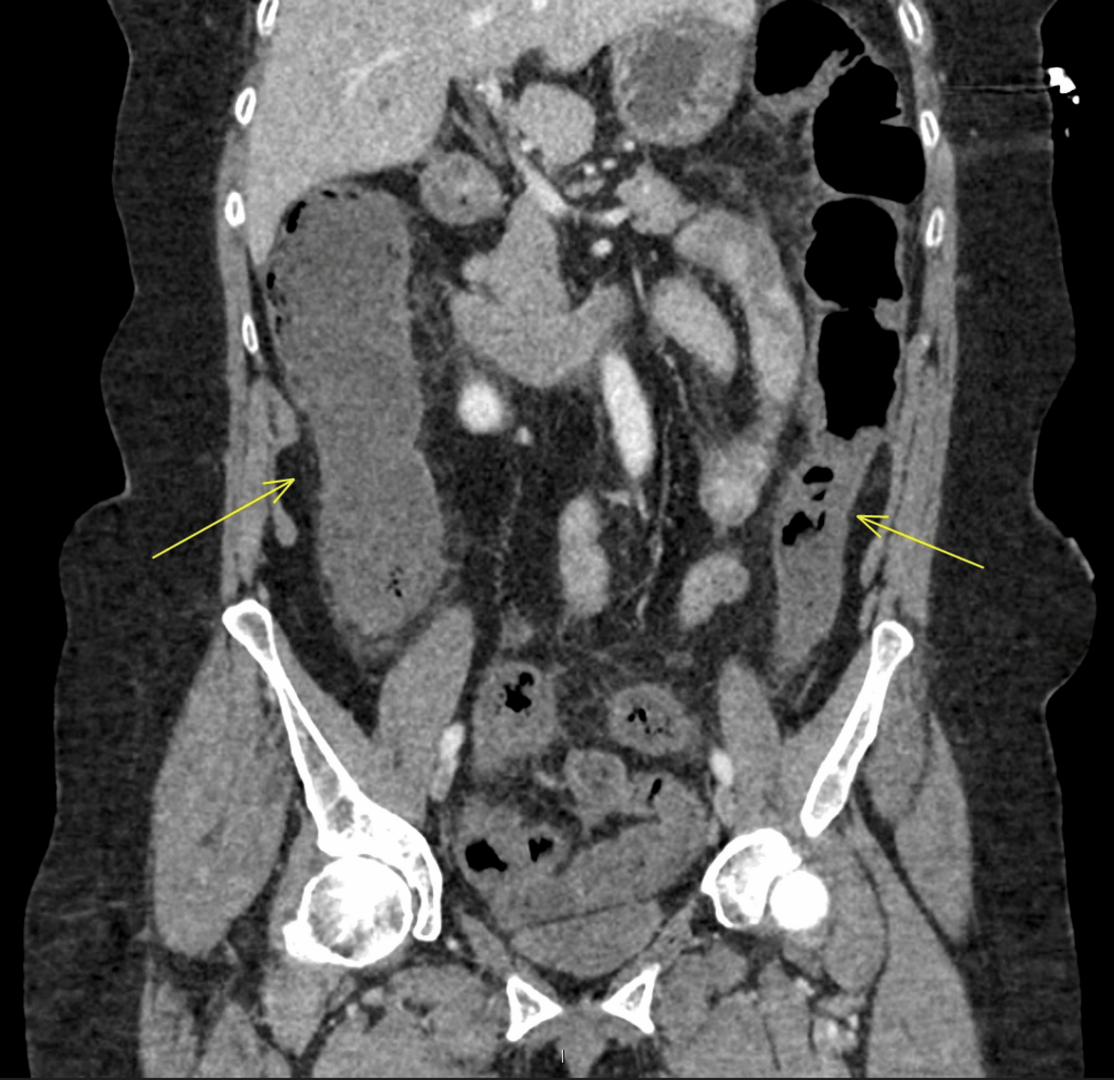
Coronal view of abdominal CT scan showing fat stranding and dilated colon with reduced wall enhancement (arrows) CT: computed tomography
